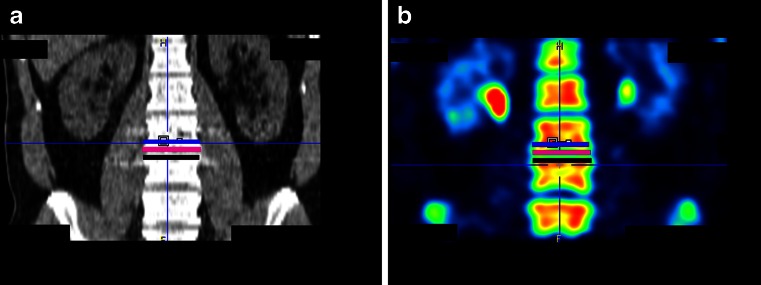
^18^F-Fluoride PET analysis of the segment operated upon. Coronal CT and PET images of the lumbar spine after posterior lumbar interbody fusion, in which three VOIs were drawn in the segment operated upon: the lower endplate of the cranial vertebra (*blue*), the intervertebral disc space (*pink*) and the upper endplate of the caudal vertebra (*black*)
